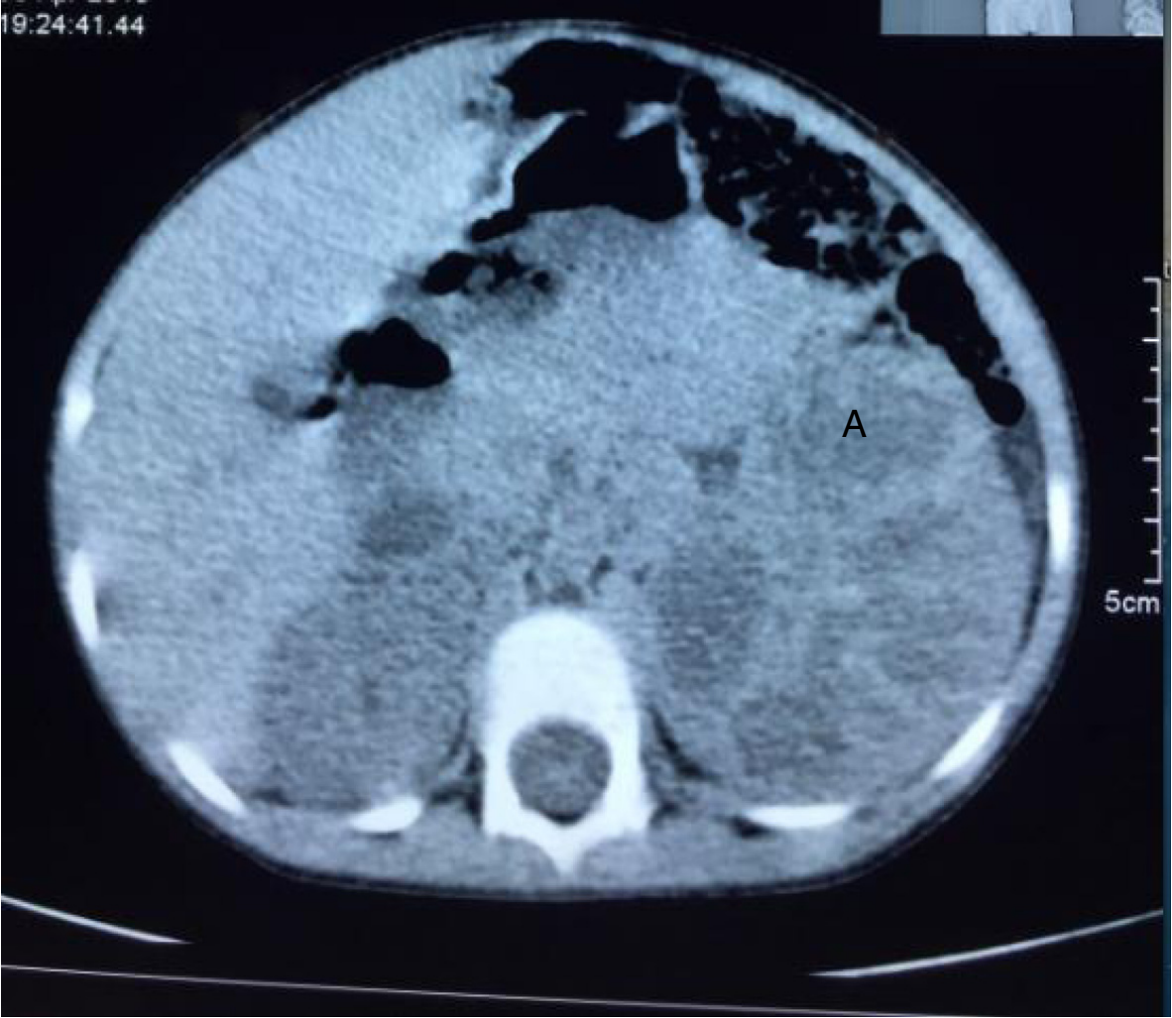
Plain abdominal CT-scan showing an enlarged left kidney with multiple cystic components (A) and calcifications
